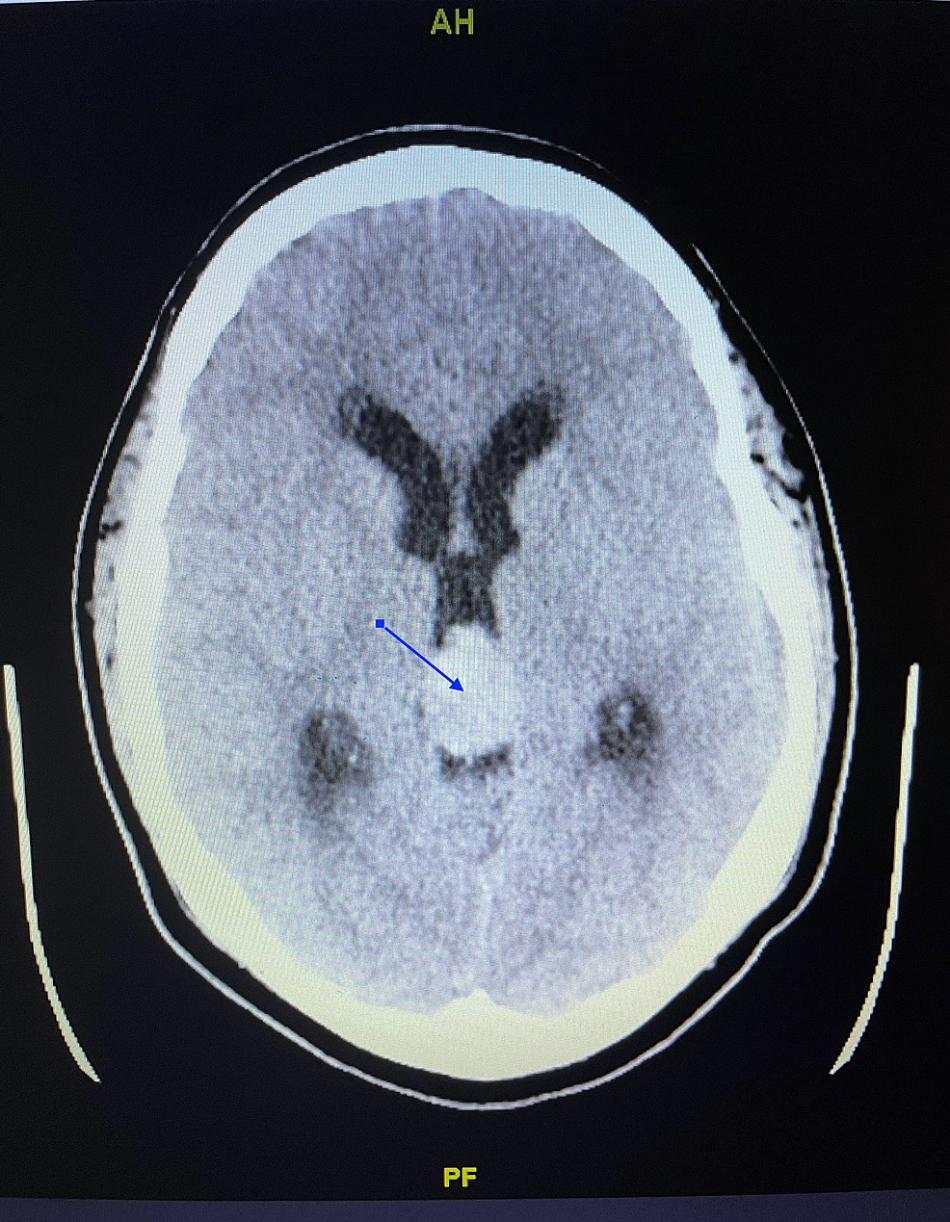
CT scan demonstrating pineoblastoma (arrow). CT: computed tomography
